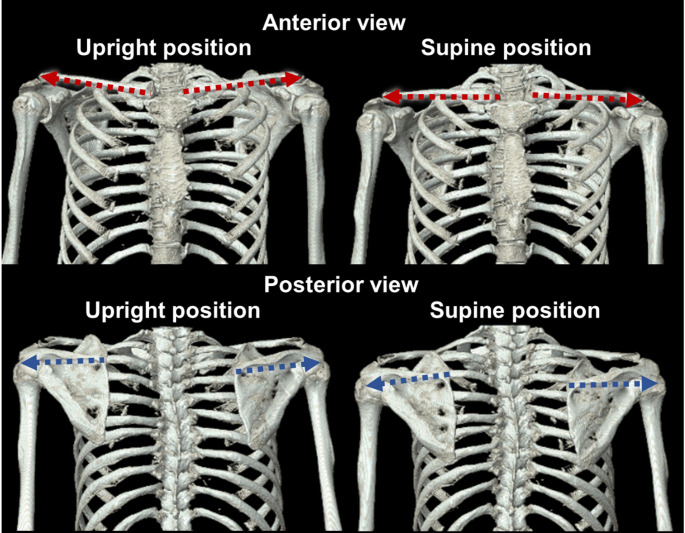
Volume-rendered CT images obtained in the supine (left) and upright (right) positions. Compared with the supine position, clavicular elevation (red dotted lines) and scapular upward rotation (blue dotted lines) are reduced in the upright position due to gravitational load
